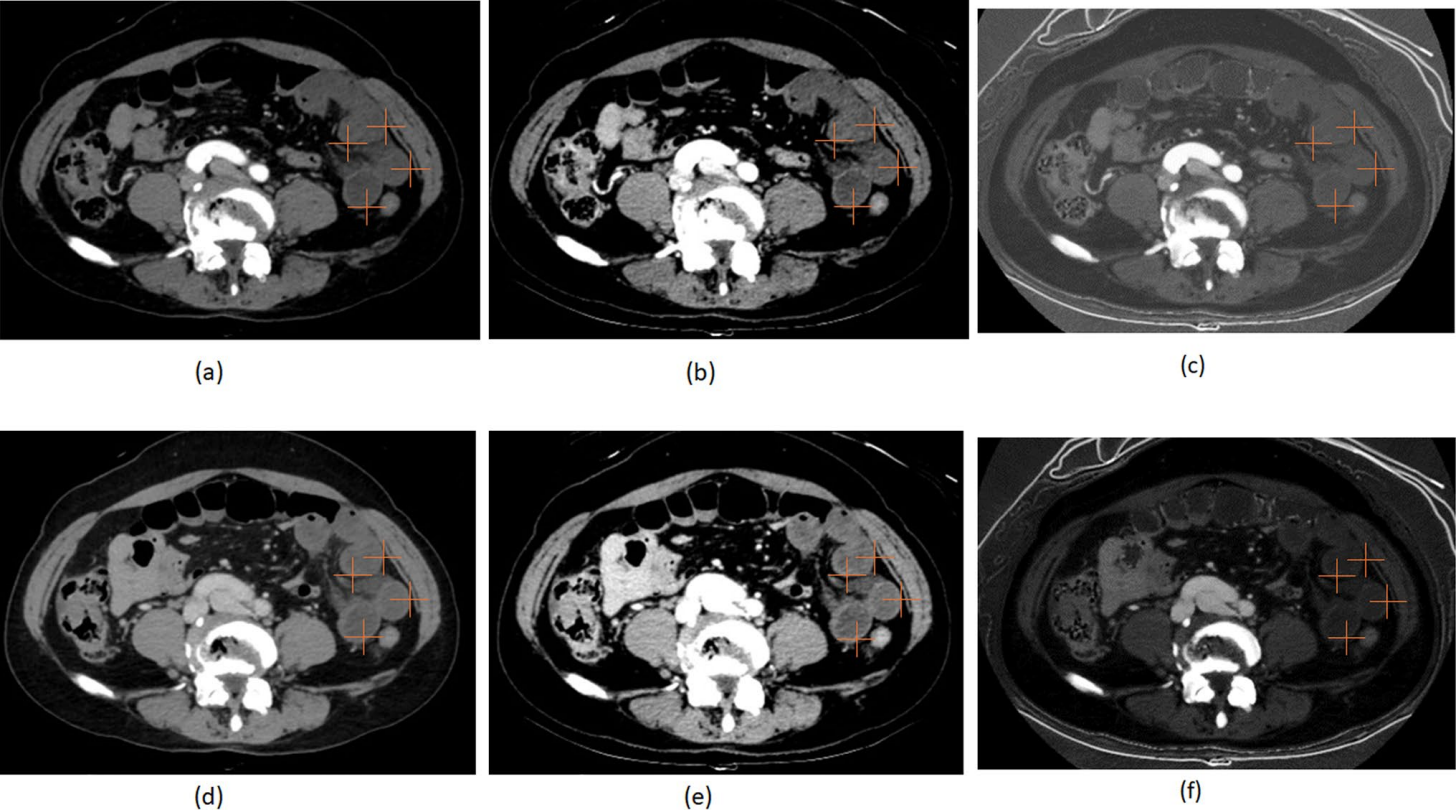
Representative images from a 54-year-old woman with surgically confirmed acute small bowel ischemia (ASBI) who required resection of 50 cm of small bowel due to necrosis. The two radiologists independently placed ten cursors on the small bowel wall in areas appearing to have reduced contrast enhancement in the arterial phase (a–c 70-keV, 40-keV, iodine quantity) and venous phase (d–f 70-keV, 40-keV, iodine quantity), ensuring that the cursors were placed at the same anatomical locations on the 70-keV images, 40-keV images, and iodine maps within each phase. In this slice, four cursors are shown. The mean CT values in the arterial phase were 27.0 HU at 70-keV and 51.3 HU at 40-keV, with a mean iodine quantity of 4.0 μg/cm^3^. In the venous phase, the mean CT values were 35.7 HU at 70-keV and 53.4 HU at 40-keV, with a mean iodine quantity of 4.0 μg/cm^3^
